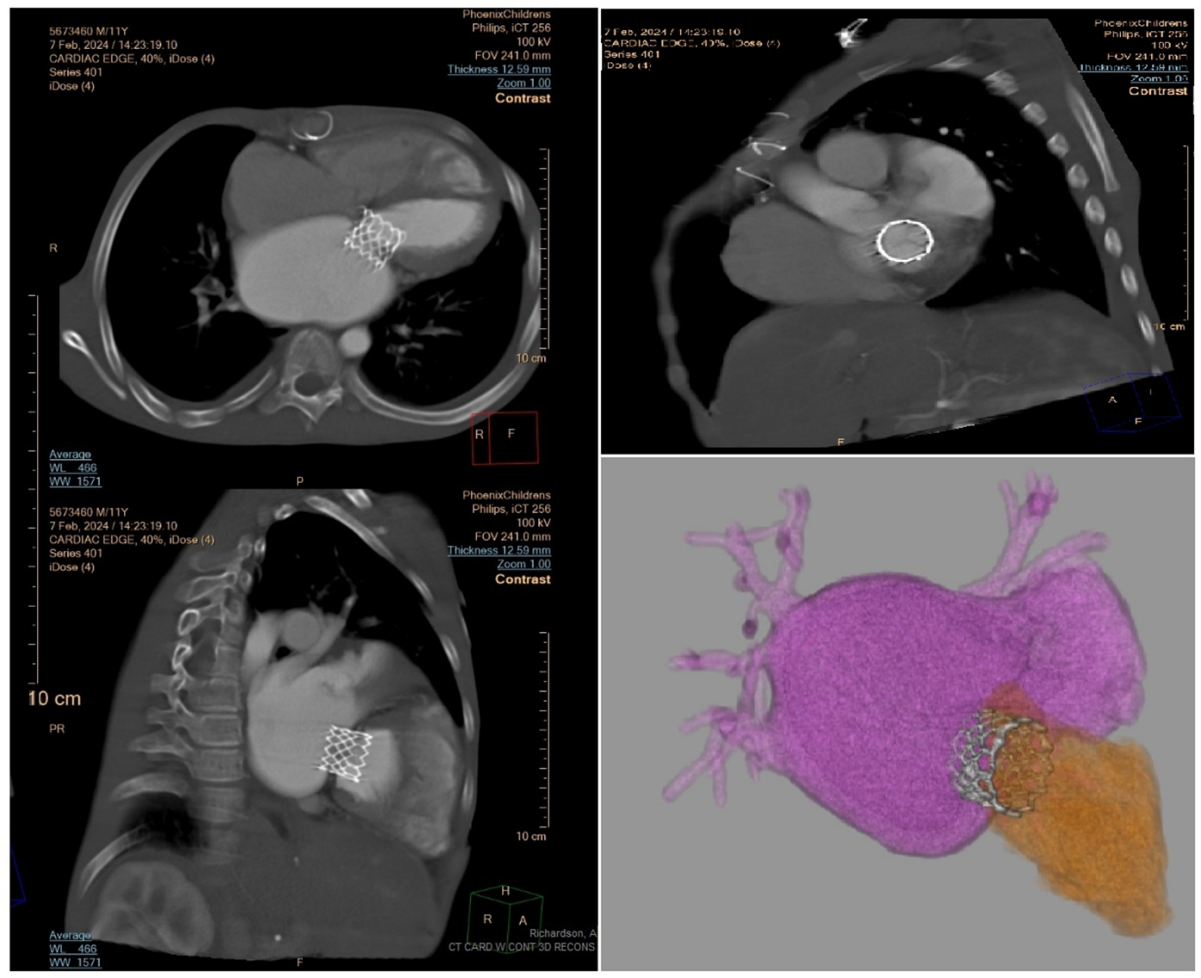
Computed tomography multiplanar reformation, cross-sectional and 3-dimensional models of implanted stent-based mitral valve at postoperative follow up.
